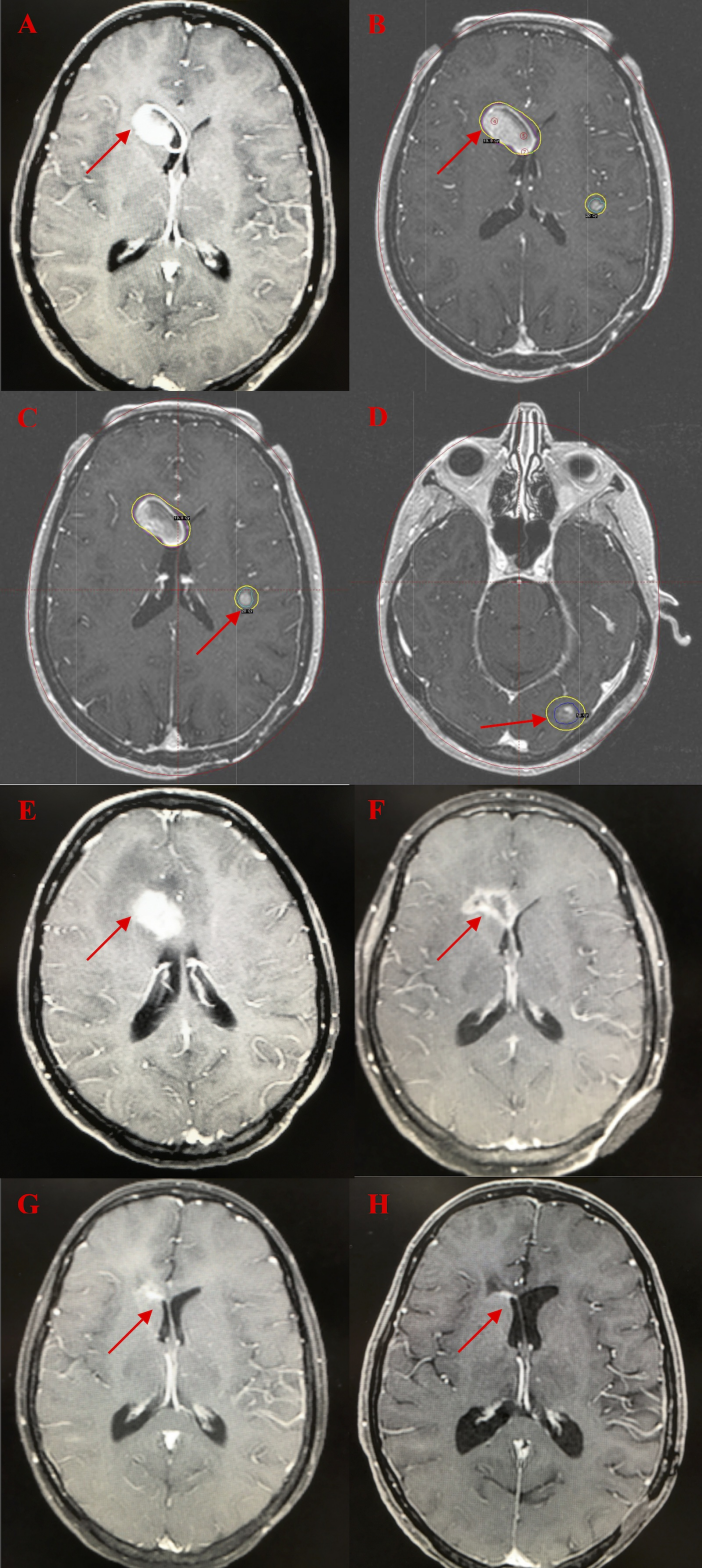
Serial Brain MRI Images 1A: Initial magnetic resonance imaging (MRI) shows the largest of three brain metastases, 12-06-2006 1B: Gamma Knife plan for right frontal brain metastasis, 12-13-2006 1C: Gamma Knife plan for left frontal brain metastasis, 12-13-2006 1D: Gamma Knife plan for left occipital brain metastasis, 12-13-2006 1E: MRI 9 months after Gamma Knife shows marked radiation necrosis and edema, 9-26-07 1F: MRI 18 months after Gamma Knife shows resolving radiation necrosis, 5-23-2008 1G: MRI 21 months after Gamma Knife shows minimal residual enhancement, 10-23-2008 1H: MRI 10.7 years after Gamma Knife shows no evidence of disease, 8-02-2017
